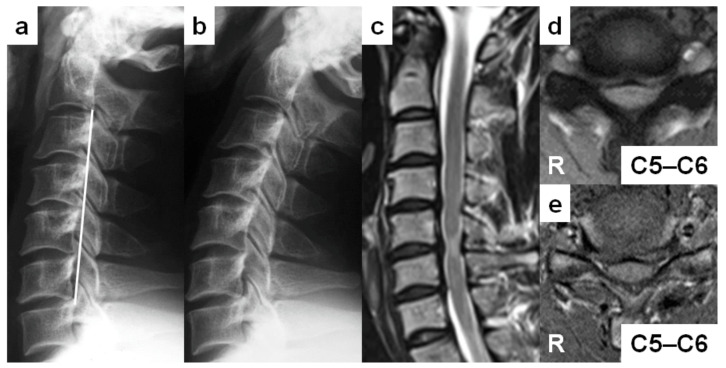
Report of a 27-year-old man with cervical spondylotic myelopathy (case no. 1). (a,b) Lateral cervical spine radiographs in neutral (a) and full-extension (b) positions demonstrating the “straight” cervical sagittal alignment. (c) A sagittal T2-weighted magnetic resonance image (MRI) demonstrating “degenerative” discs from C3–C4 to C6–C7, spinal cord compression from C4–C5 to C6–C7, and an intramedullary increased signal intensity lesion at C5–C6. (d) An axial T2-weighted MRI demonstrating the narrowed cervical spinal canal with an intramedullary increased signal intensity area at C5–C6. (e) An axial T1-weighted MRI demonstrating the “angular-edged” spinal cord deformity at C5–C6.
